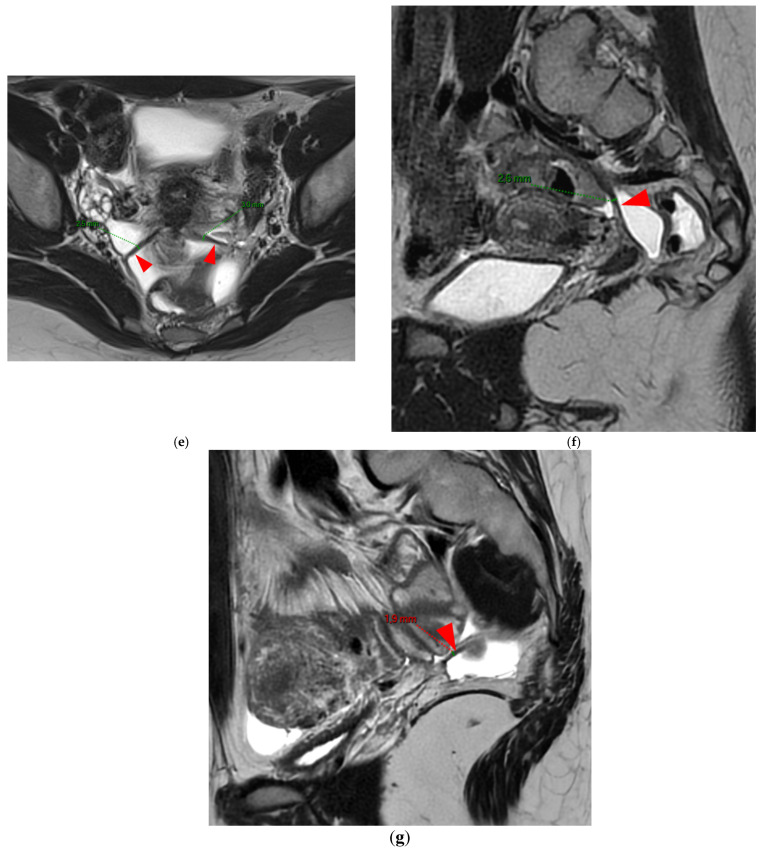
Pelvic MRI scans of seven patients with HTD type 3B USLs. (a) Sagittal T2WI: a thickened (2.1 mm) and stiffened right USL (*arrowhead*) with “bowstringing”. (b) Axial T2WI: a caliber disparity (*dashed arrows*) with focal thickening (4.2 mm) of the left proximal USL (*arrowhead*). (c) Sagittal T2WI: a caliber disparity (*dashed arrows*) with focal thickening (2.5 mm) of the right distal USL. (d) Axial T2WI: a right USL with a notched and irregular surface (*dashed arrows*). (e) Axial T2WI: thickened and stiffened left (3 mm) and right (2.5 mm) USLs with “bowstringing” of both USLs (*arrowheads*). (f) Sagittal T2WI: a thickened (2.6 mm) right USL with a stiffened appearance characterized by a steep vertical orientation (*arrowhead*). (g) Sagittal T2WI: the right USL appears thin (1.9 mm) but stiffened (*arrowhead*), exhibiting “bowstringing”. These findings led to its reclassification from type 2 to type 3B.
